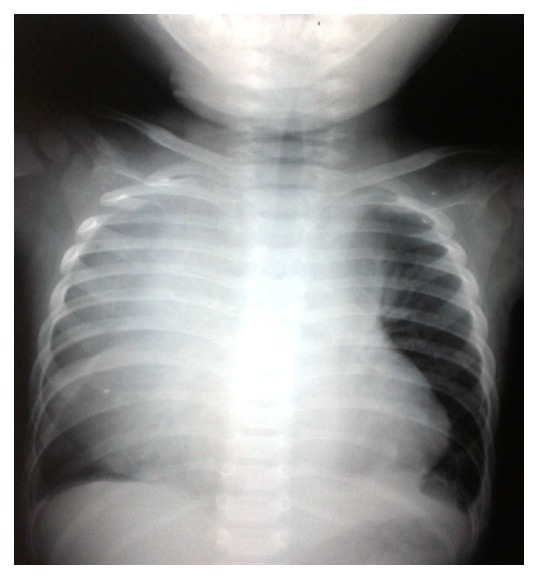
Chest radiography showing large homogeneous mediastinal opacity with mediastinal enlargement.
